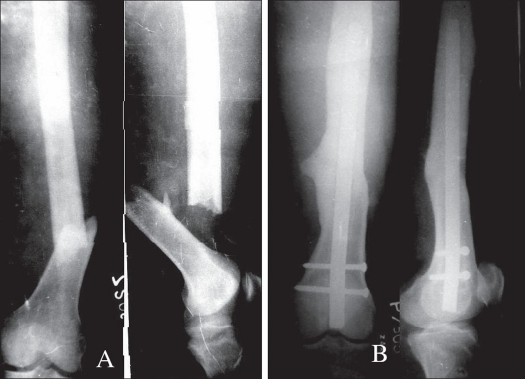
A) Anteroposterior and lateral radiograph of a patient having compound Type IIIB supracondylar fracture femur fixed with intramedullary supracondylar nail after 13h of injury. B) Showing good union after 23 weeks
